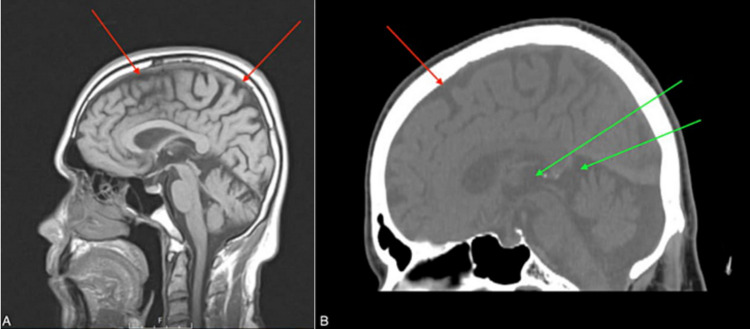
Sagittal T1 diffusion-weighted head MRI. (A) Hypoattenuation in the cortex suggests sulci enlargement and generalized cortical atrophy (red arrows). (B) Further evidence of cortical volume loss (red arrow) and concomitant ventricular enlargement (green arrows)
